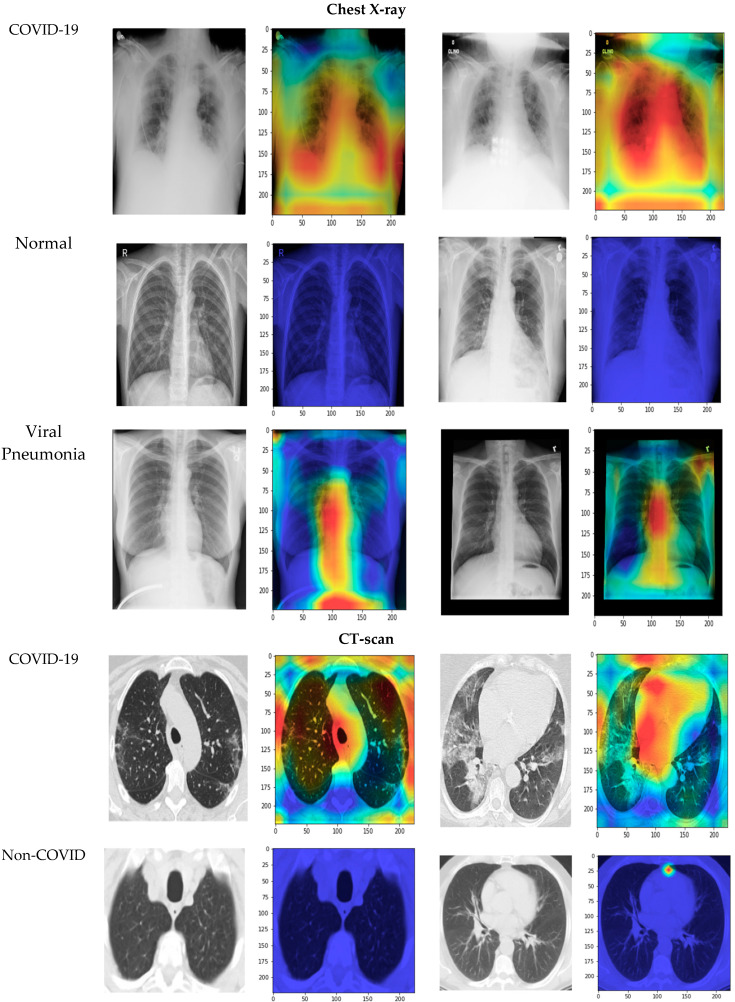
Grad-CAM sample results for COVID-19, normal, and viral pneumonia classes in the chest X-ray dataset and COVID-19 and non-COVID classes in the CT scan dataset.
